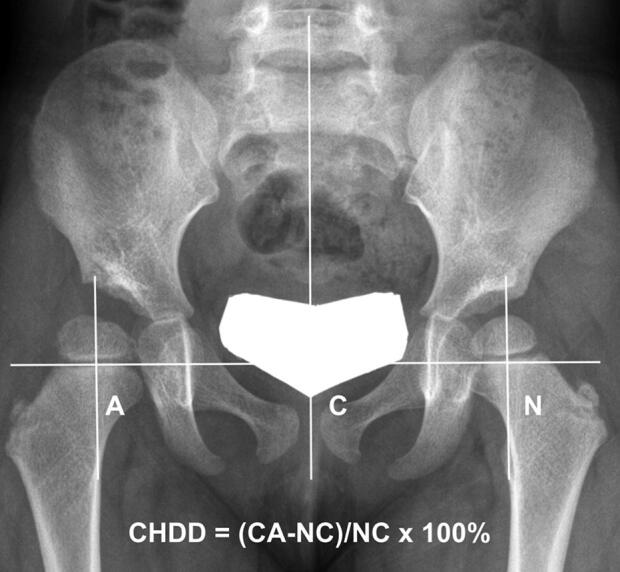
Measurement of center–head distance discrepancy (CHDD) on anteroposterior radiograph of the hip. The CHDD was defined as the difference in the center–head distance between the DDH side and the normal side, and expressed as a percentage of the normal side measurement.
